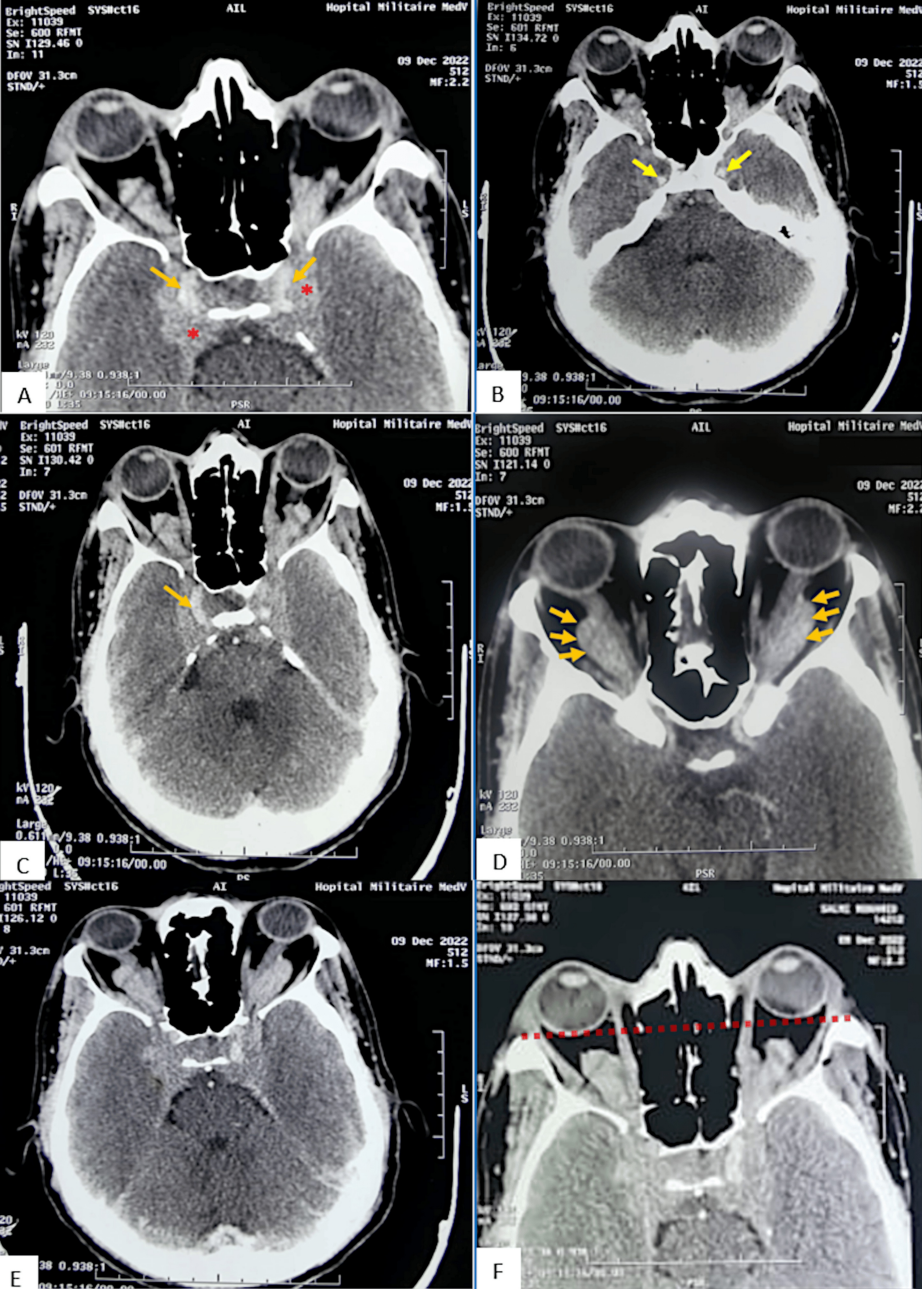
Axial CE brain CT. The CT scan revealed a 47x25 mm moderately enhancing lesional mass centered on the spheno-cavernous region (red asterisk, A, E), heterogeneous in appearance, with the invasion of the sheaths of both optic nerves (orange arrowhead, A, C, D), the superior orbital fissures, peri-nerve sheaths, and laterally into the cavernous sinuses, encompassing both carotid arteries in the intra- and supracavernous portions (yellow arrowhead, B). Grade III bilateral exophthalmos is indicated by the dotted line (F). CE, contrast‐enhanced; CT, computed tomography
